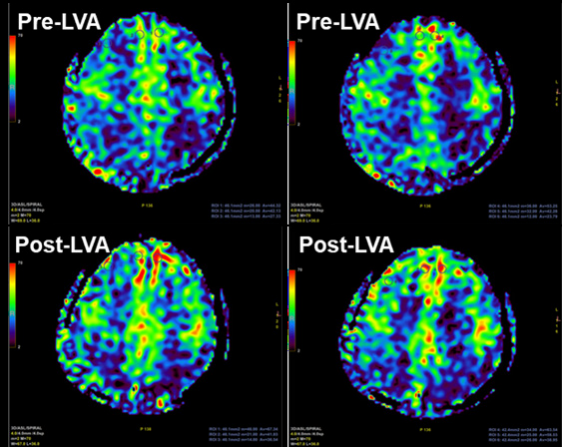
Lymphatic-venous anastomosis improved brain perfusion in Alzheimer’s disease. Cerebral perfusion in an Alzheimer’s disease patient was enhanced 7 days after undergoing lymph–venous anastomosis, as detected and quantified using arterial spin labeling magnetic resonance imaging with spiral acquisition on a three-dimensional scanner. Sourced from Dr. Mian Guo’s research team.
